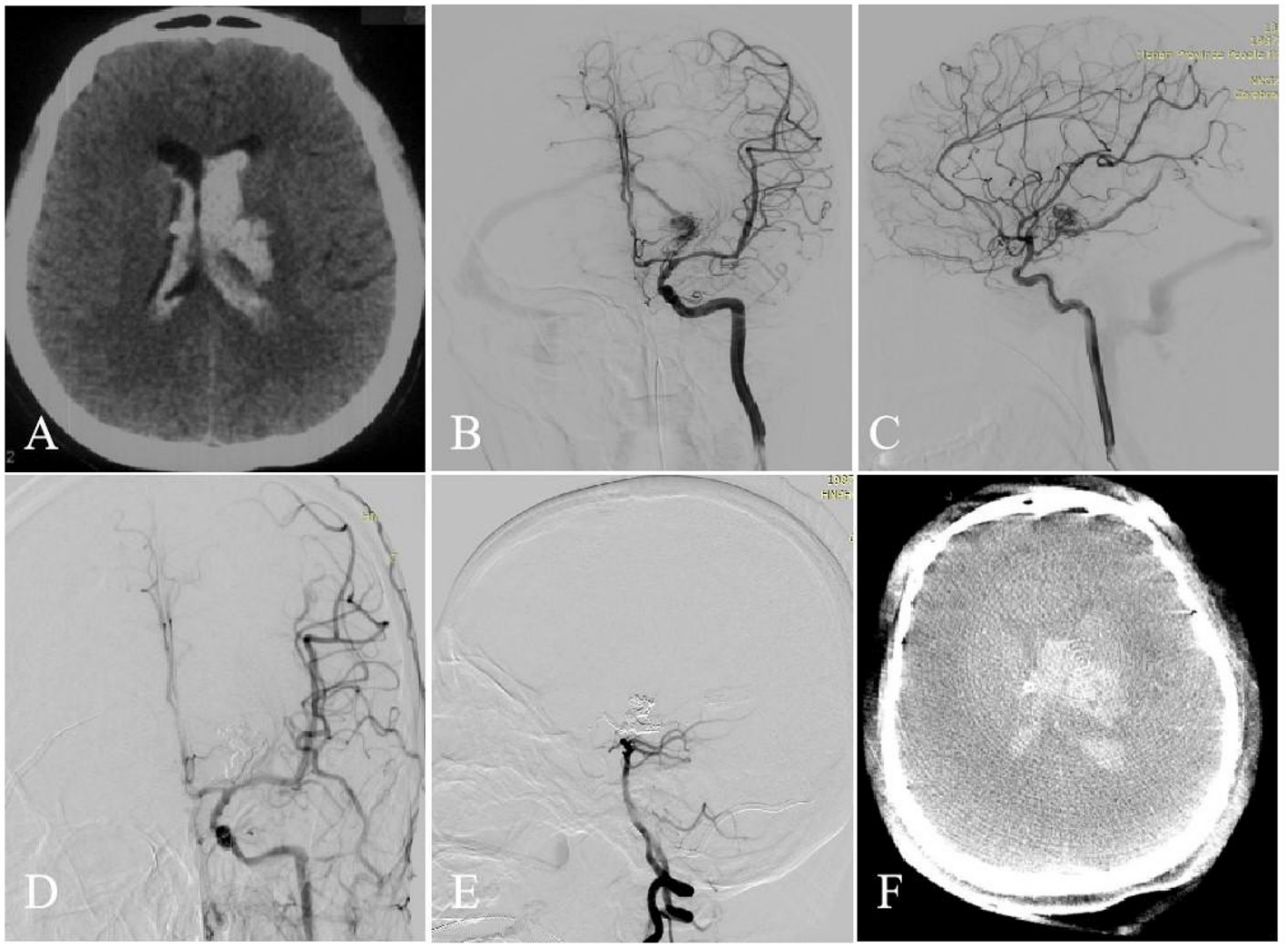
A 31-year-old woman with left basal ganglia AVM. Intraventricular hemorrhage occurred (A, CT). Selective DSA of the left ICA [anteroposterior (B) and lateral (C) views] demonstrated that the AVM is fed by the ACA, AcrA, and PCA and drained a single venous outlet via the VG. At the end of the operation, the AVM did't appear at the last angiography [anteroposterior (D) and lateral (E) views]. Intraoperative intraventricular hemorrhage occurred (F, Dyna CT).
